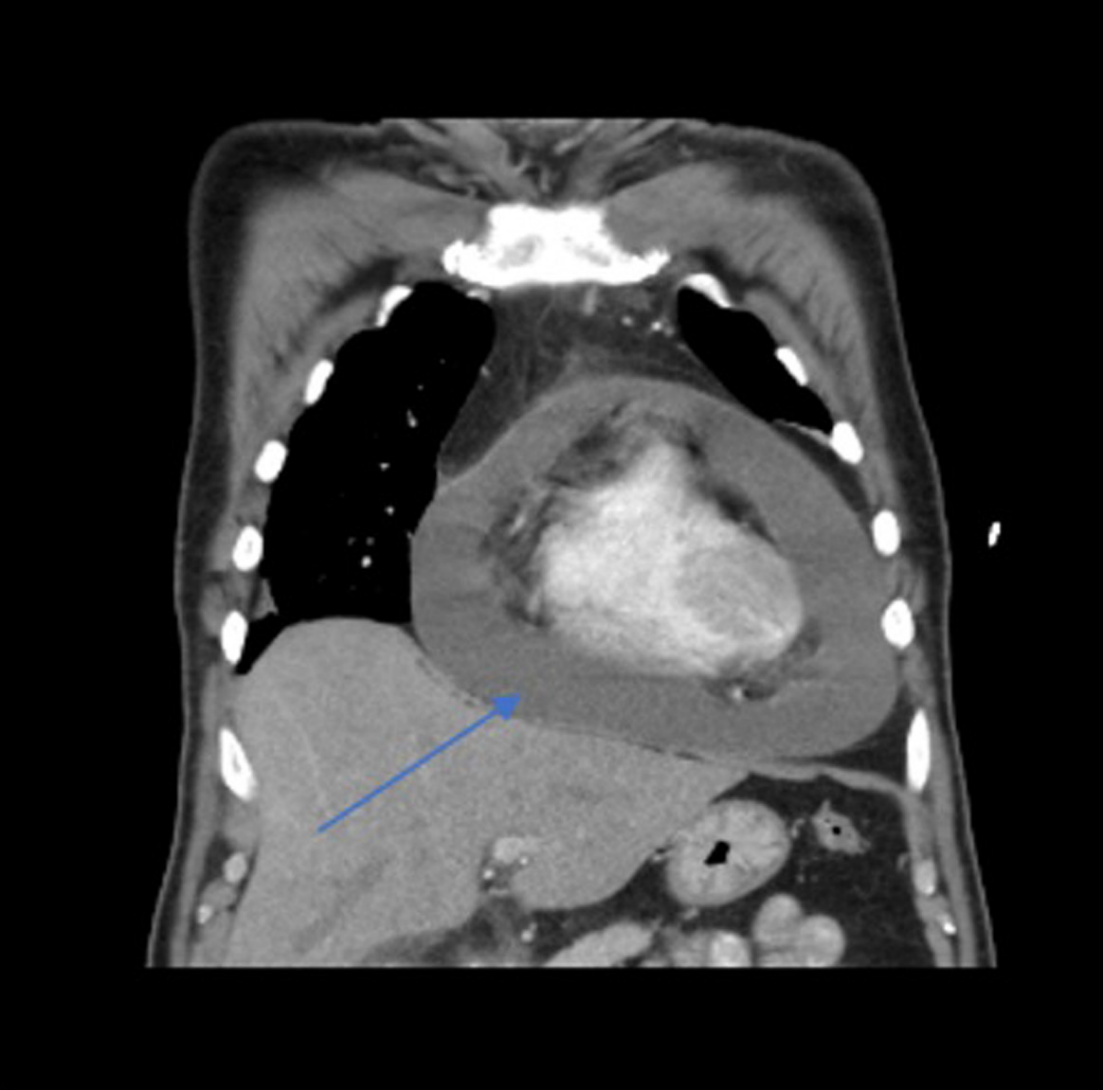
Coronal view: CT scan of the chest showing large pericardial effusion (blue arrow).
